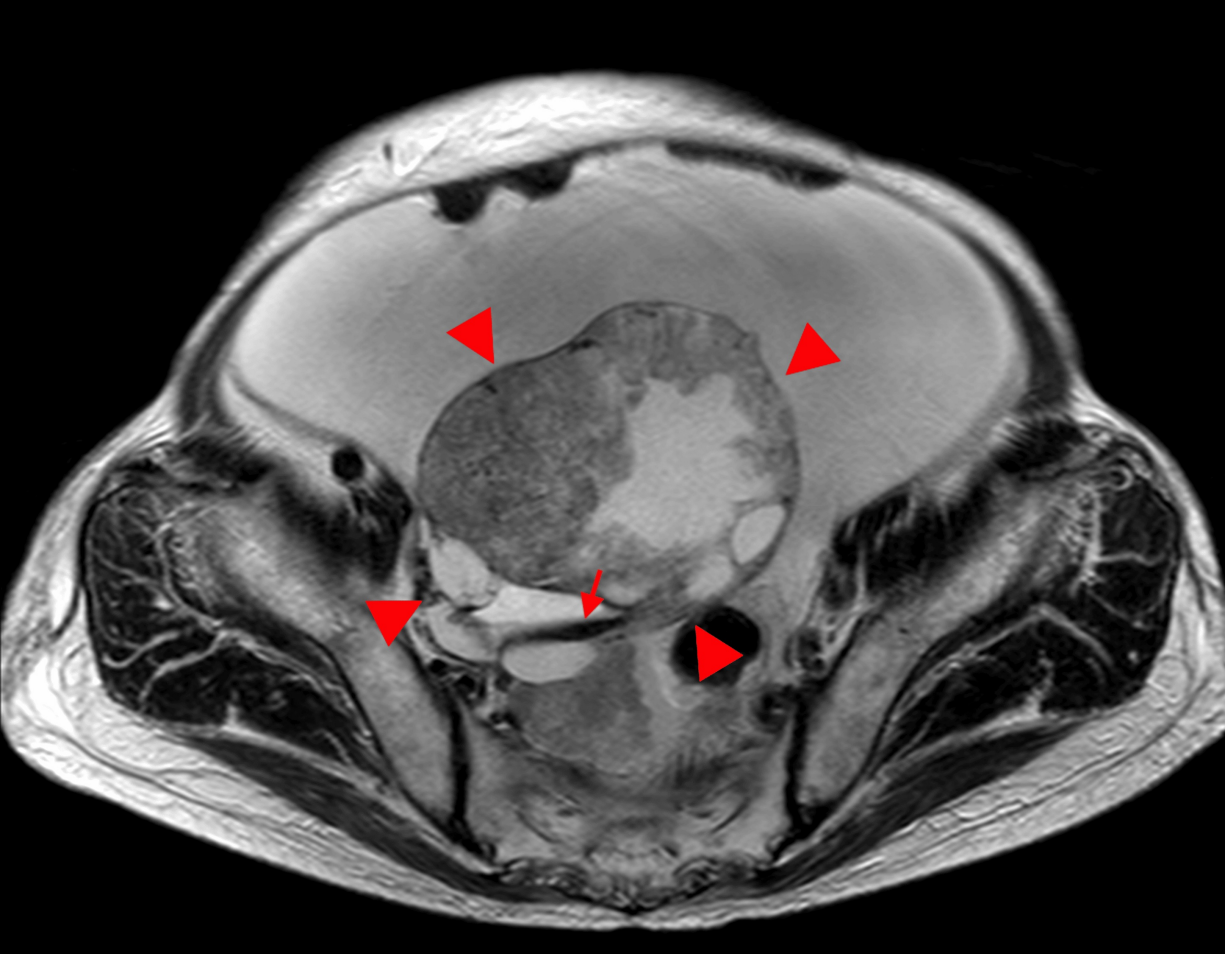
MRI in a 77-year-old woman with ovarian carcinosarcoma of the right ovary A large tumor measuring up to 12 cm in diameter is observed, consisting of broad-based, concentric solid components with heterogeneous signal intensity on the axial T2-weighted image and associated cystic components (arrowhead). The posterior margin is irregular and contains a fluid-fluid level suggestive of hemorrhage (arrow).
